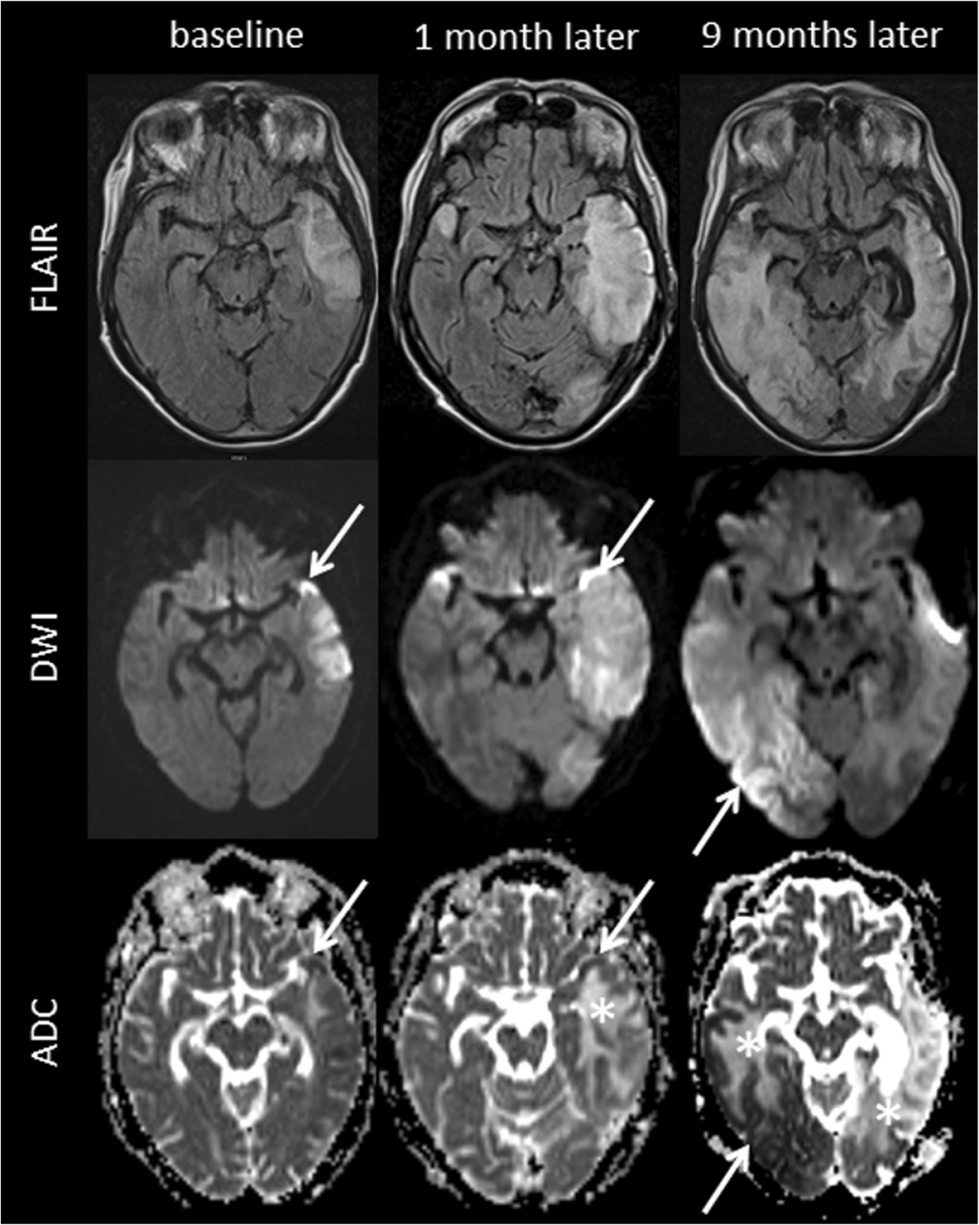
MR imaging findings. Fluid attenuated inversion recovery (FLAIR), diffusion weighted imaging (DWI), and apparent diffusion coefficient (ADC) images at first presentation and until 9 months later are presented. FLAIR images demonstrate step-wise progressing FLAIR hyperintense oedema not corresponding to a vascular territory resulting in a local mass effect. DWI delineates both, i) cortical areas of restricted diffusion that are hyperintense on DWI and hypointense on ADC (arrows), as well as ii) subcortical vasogenic oedema indicated by ADC hyperintense areas (asterisks)
